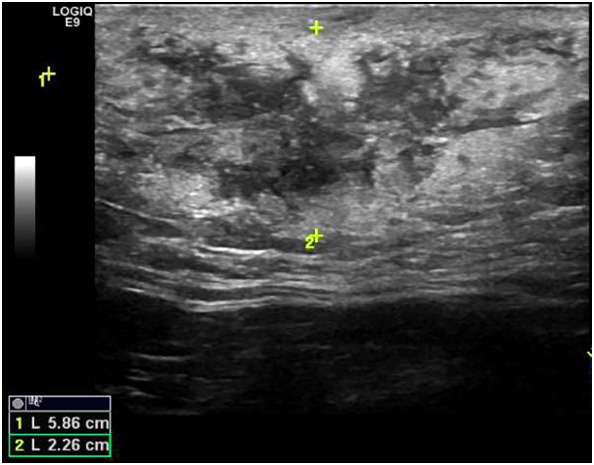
Ultrasound image of a mixed echogenic lesion in the upper outer quadrant of the right breast (5.9×5.1×2.3 cm), with indistinct margins, skin proximity, and abundant vascularity.
